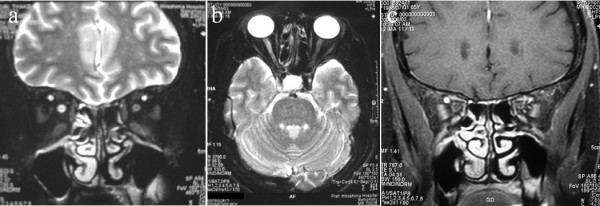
Fat-suppressed T2-weighted images of Case 2. Coronal image (a) and axial image (b) from a 66-year-old Japanese woman show high-intensity areas in the right optic nerve sheath. Fat-suppressed T1-weighted post-contrast coronal image (c) shows high-intensity areas in the right optic nerve sheath. The optic nerve sheath is enlarged and enhanced on both sides.
